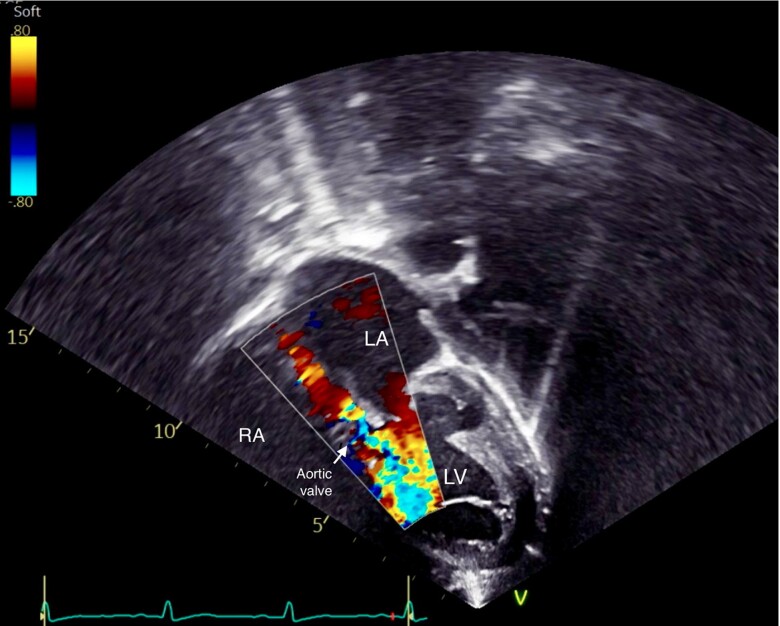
Transthoracic echocardiography images showing massive aortic valve regurgitation on five-chamber views with colour Doppler.
